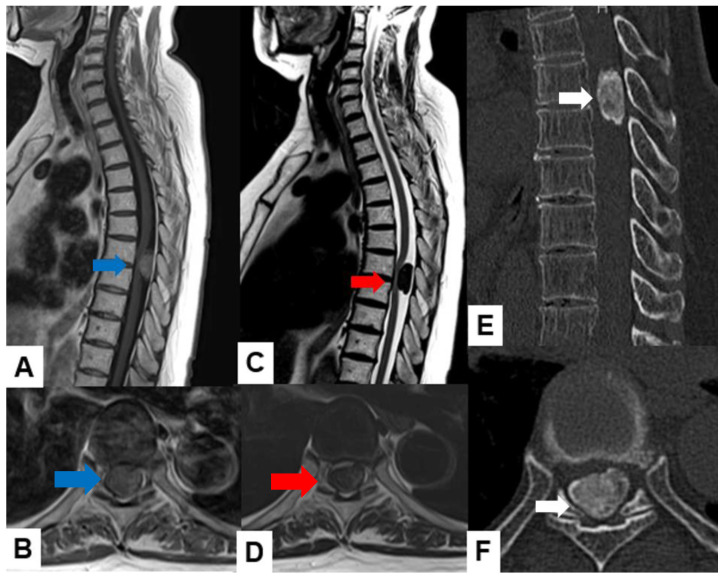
Sixty-eight F, spinal meningioma. (A) Enhanced T1-weighted midsagittal image, (B) enhanced T1-weighted axial image at T7/8, (C) T2-weighted midsagittal image, (D) T2-weighted axial image at T7/8, (E) midsagittal reconstruction CT, and (F) axial CT at T7/8. Red arrows indicate tumor, and blue arrows show tumor enhancement. The tumor is calcified (white arrows).
